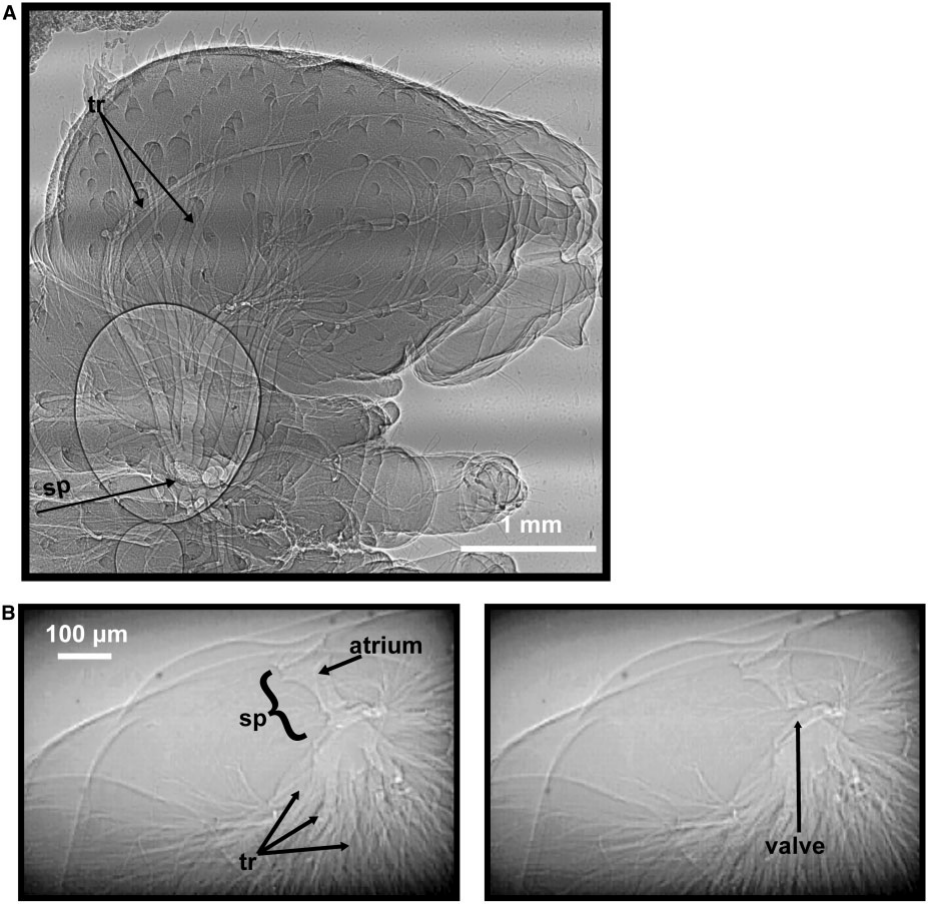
Synchrotron x-ray images of *M. sexta*, highlighting structures of the tracheal system. (A) Lateral view of a first instar hatchling’s head showing one spiracle (sp) covered by the sieve plate and numerous branching tracheae (tr). (B) Cross-sectional views of a fifth instar caterpillar, showing the spiracle, the atrium, and the muscular spiracular valve. The left panel shows the valve in the open state, and the right panel shows the valve in the closed state.
